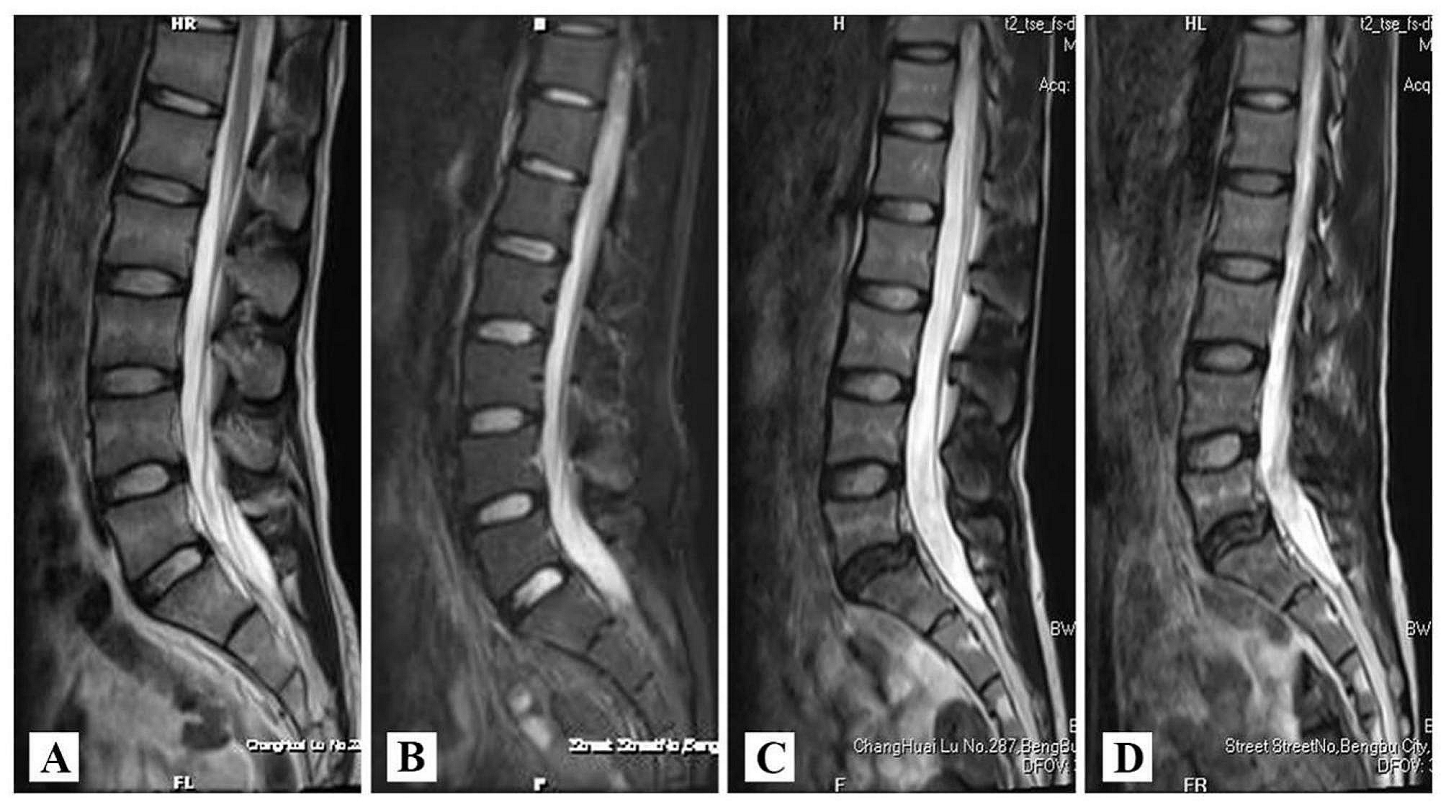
MRI indicated the Pfirrmann classification of the affected discs: (A, B) One case from grade II to grade I; (C, D) Another case from grade III to grade II
